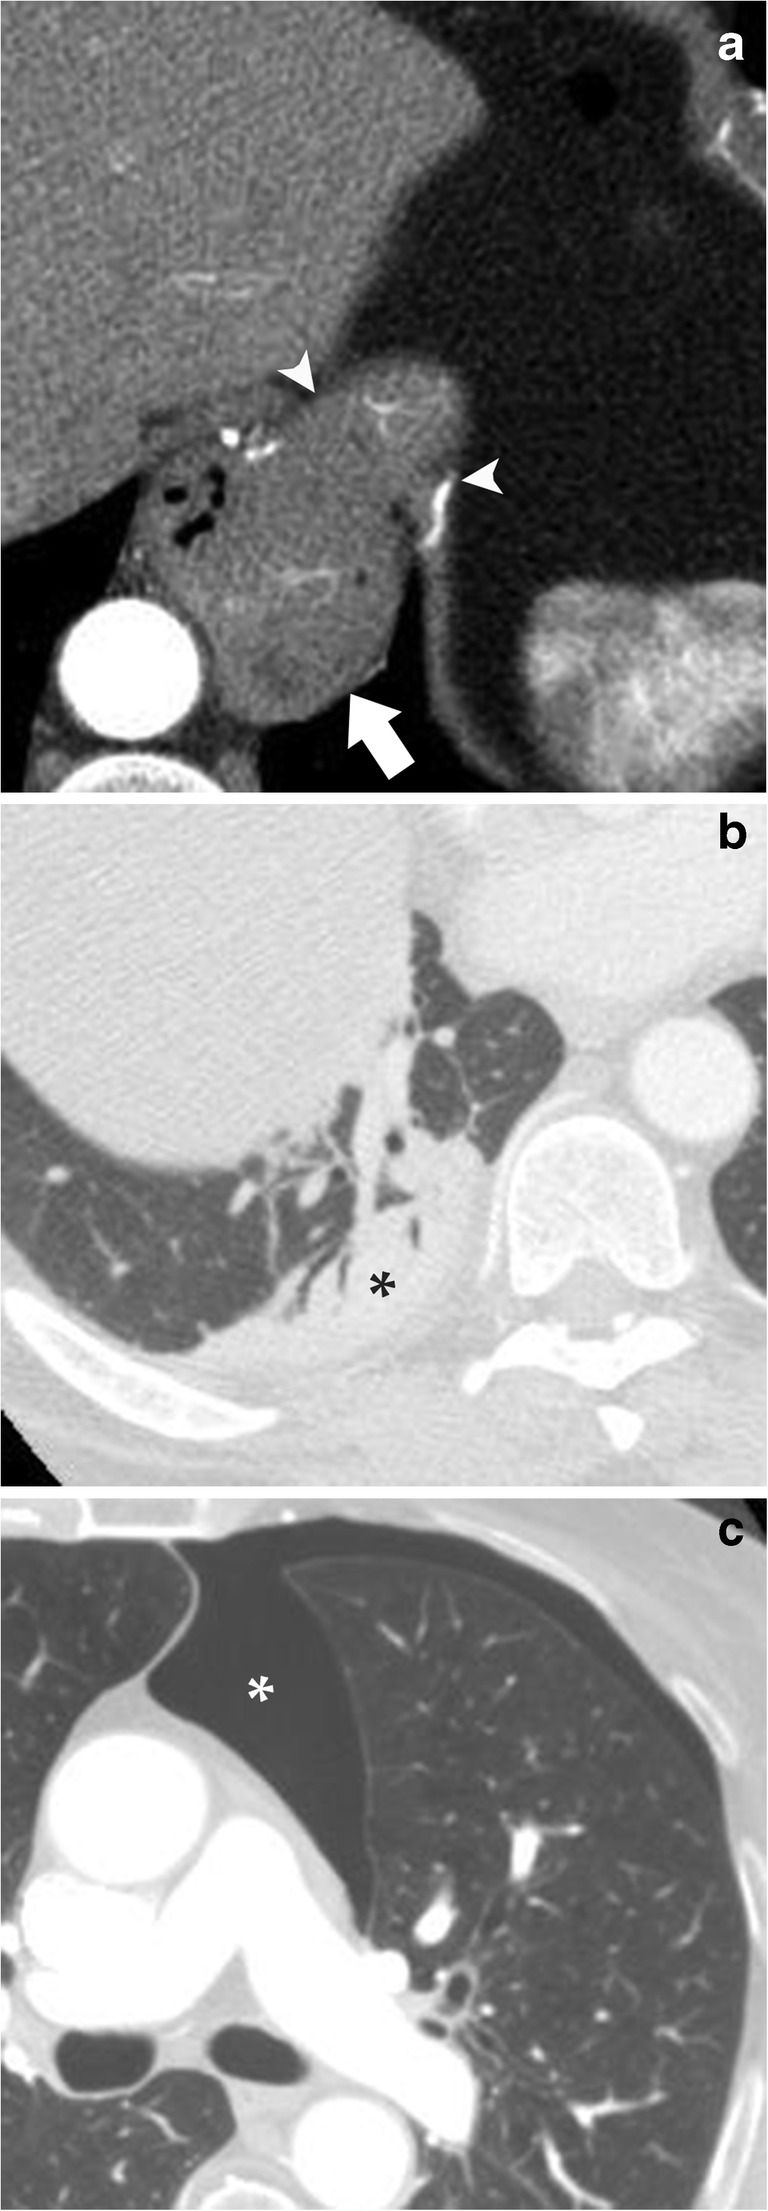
CT pure axial images showing examples of extracardiac diseases. a Axial image at the supra-diaphragmatic level showing a large hiatal hernia (*arrow*) extending into the thoracic cavity through the esophageal hiatus of the diaphragm (*arrowheads*). b Axial image with lung window, at the heart level, showing pulmonary consolidation (*asterisk*) with air bronchogram in the posterior-basal segment of the right lung. c Axial image with lung window, at the pulmonary artery bifurcation level, showing a significant anterior mainly para-mediastinal pneumothorax (*asterisk*) of the left lung
